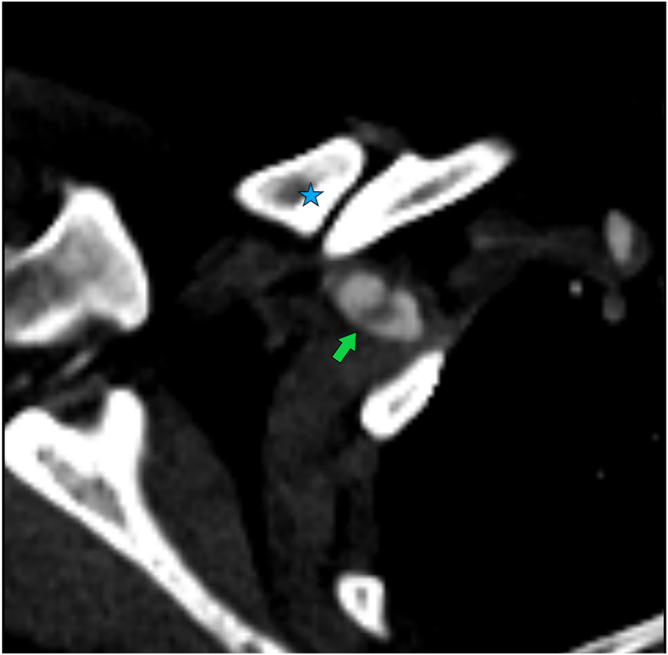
Axial CTA image showing, proximal to the thrombotic occlusion, an intimal flap inside the right subclavian artery (*greenarrow*) adjacent to the hypertrophic clavicular pseudarthrosis site (*blue star*).*CTA*, computed tomography angiography.
